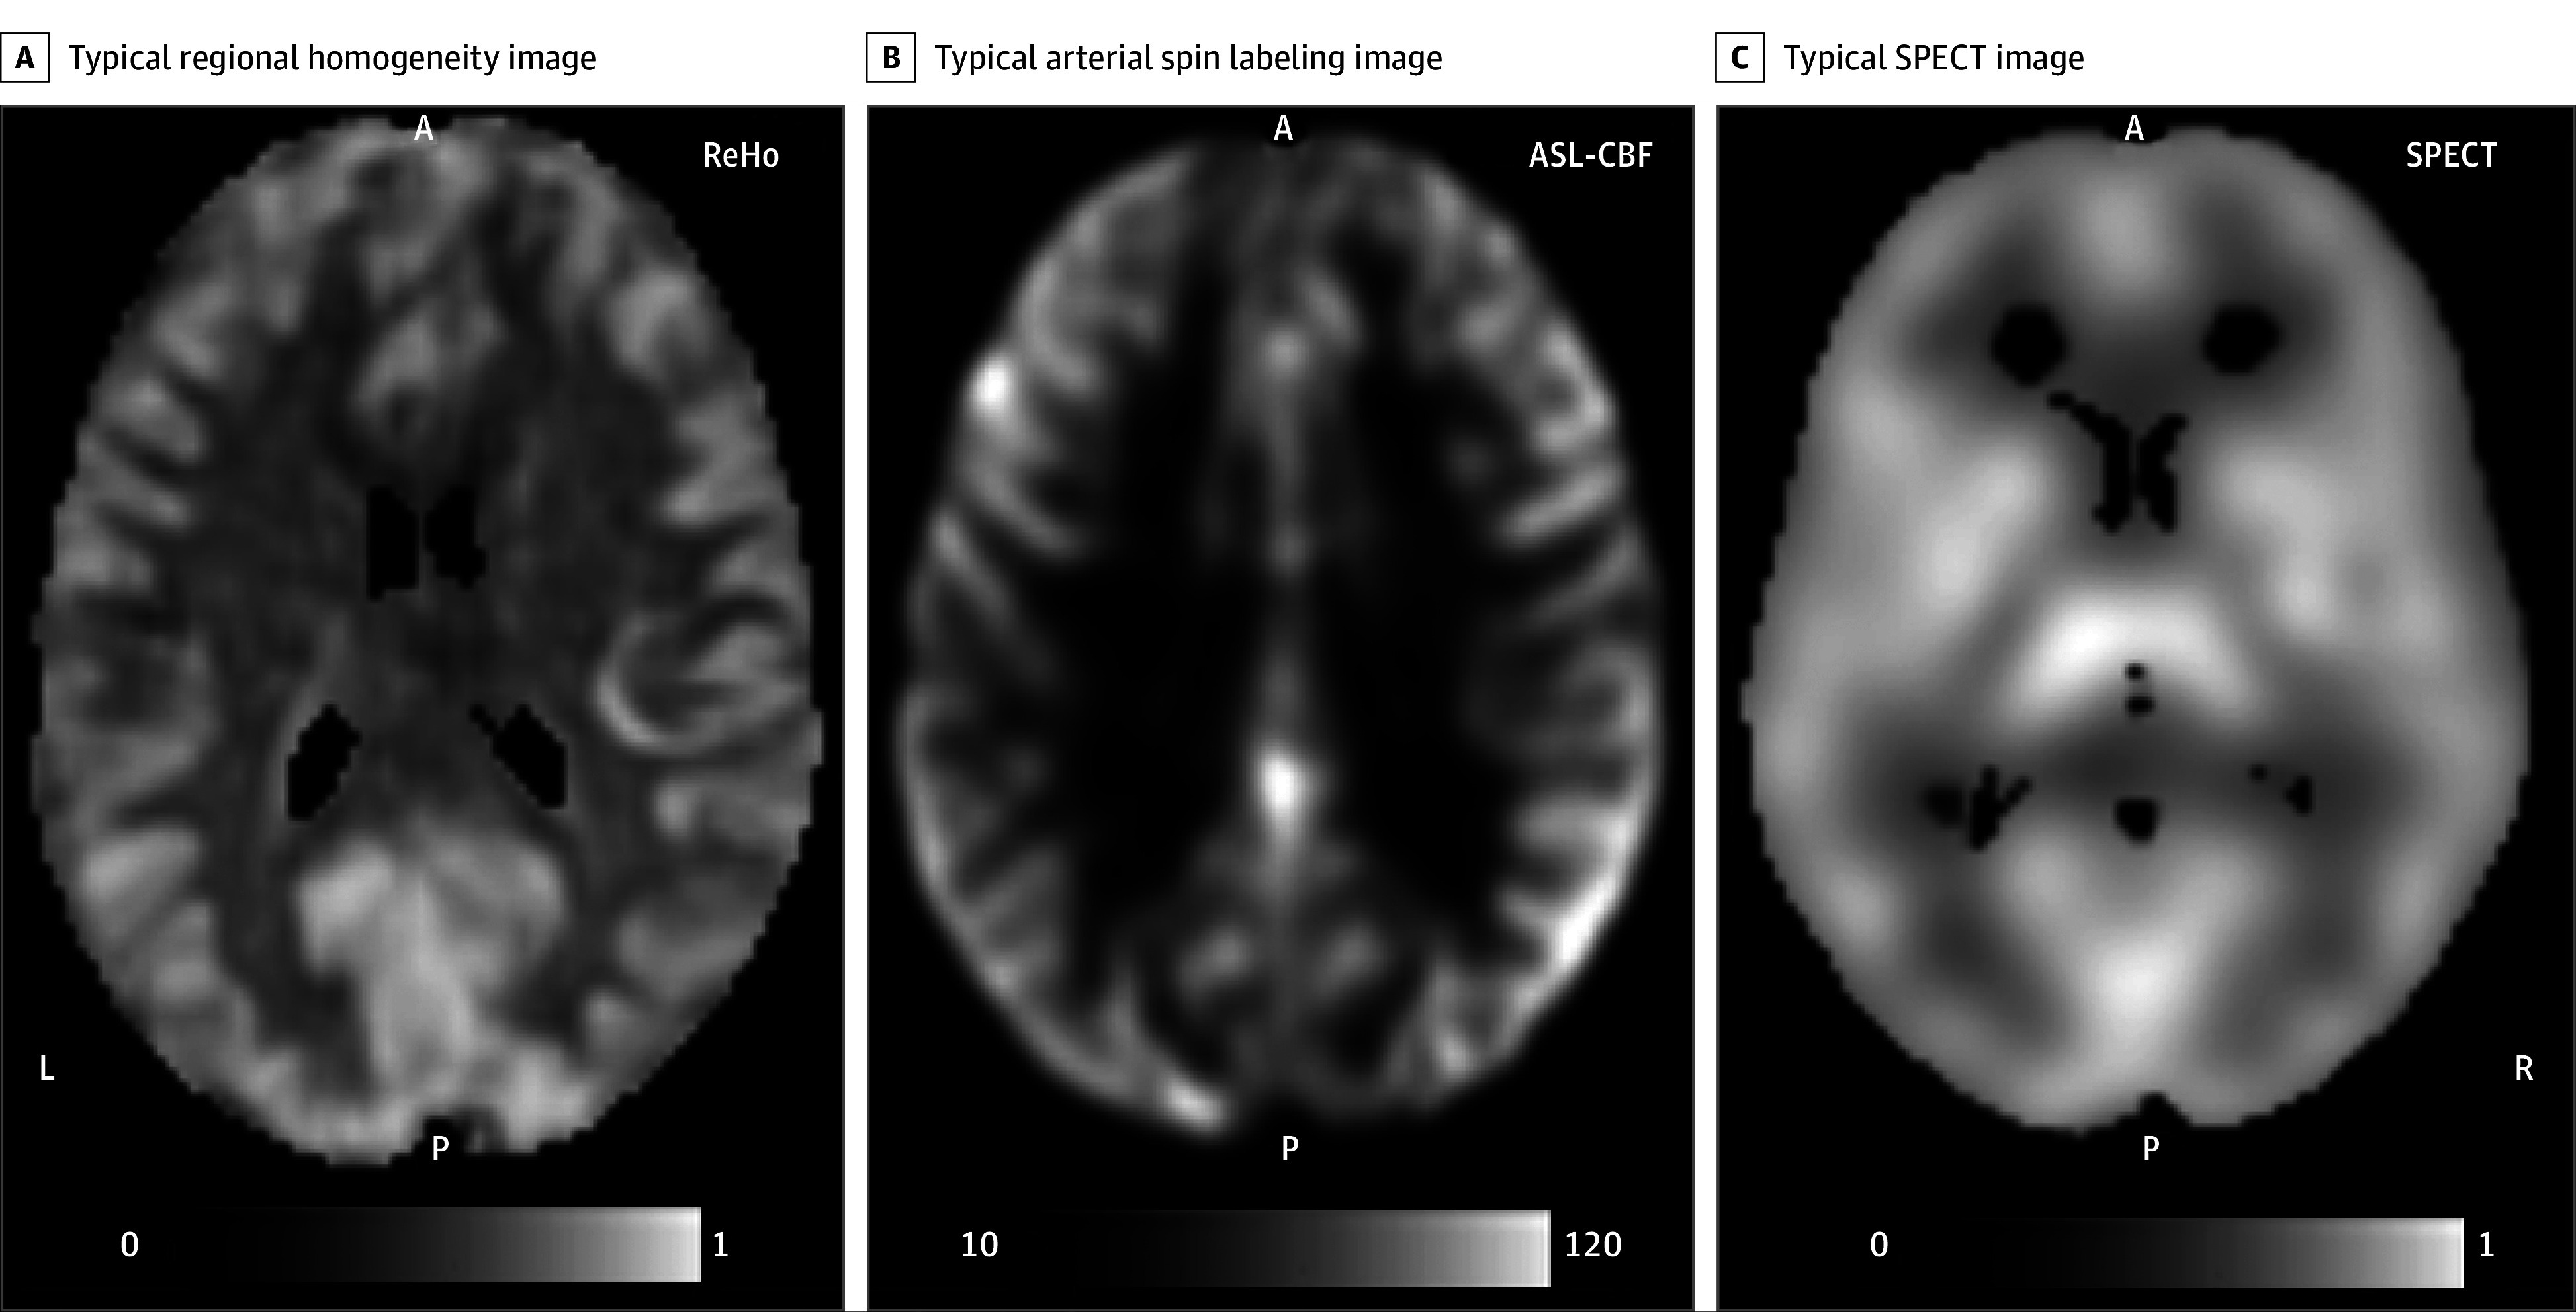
Participant Representative Maps Typical regional homogeneity (ReHo), arterial spin labeling (ASL), and single-photon emission computed tomography (SPECT) single-participant images. Despite entirely different acquisition techniques, resolutions, and scales, there are appreciable similarities between the gross ReHo signal distribution pattern and the ASL-derived cerebral blood flow (CBF) and SPECT-derived CBF patterns.
